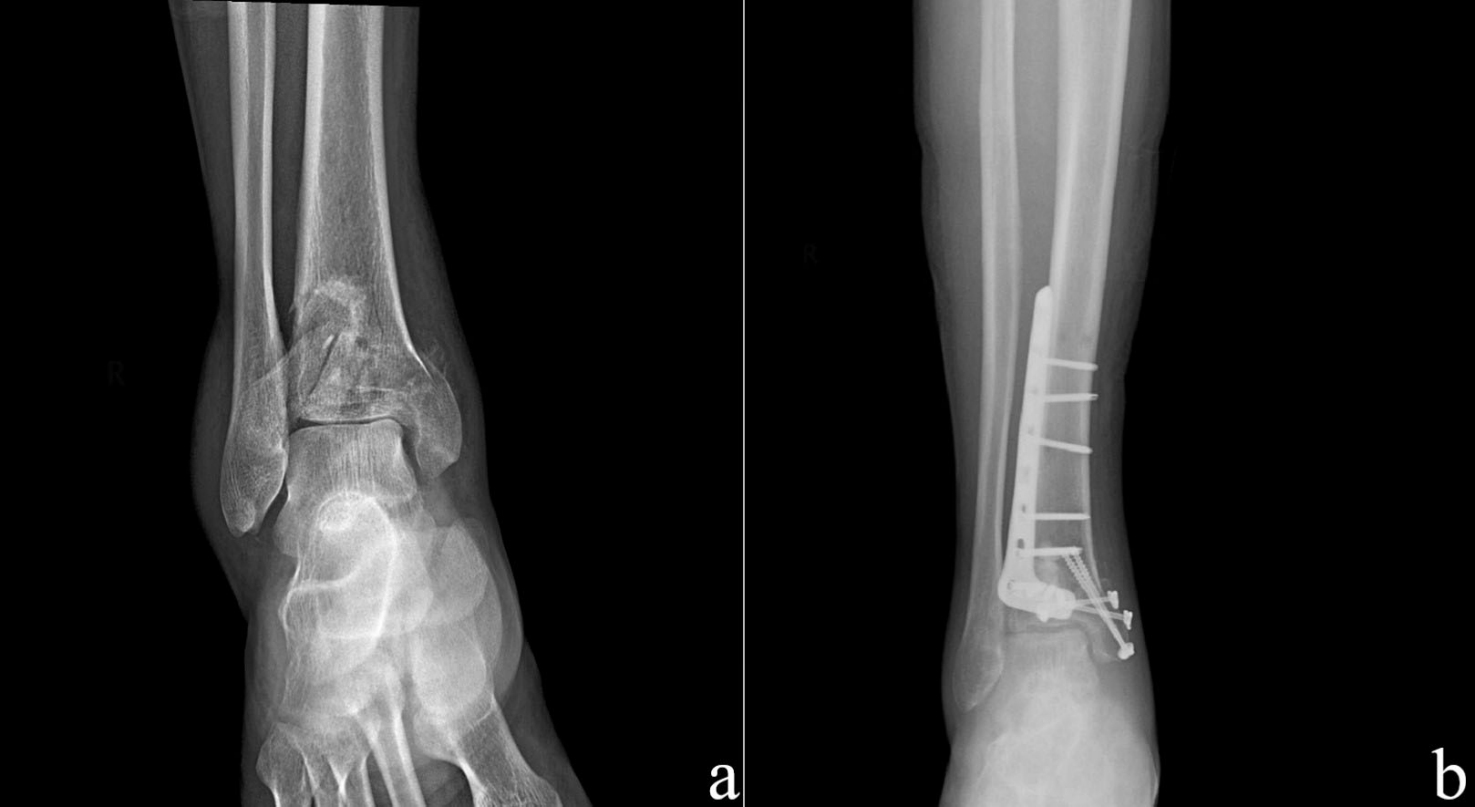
Preoperative (a) and postoperative (b) x-ray images of single step surgery.
